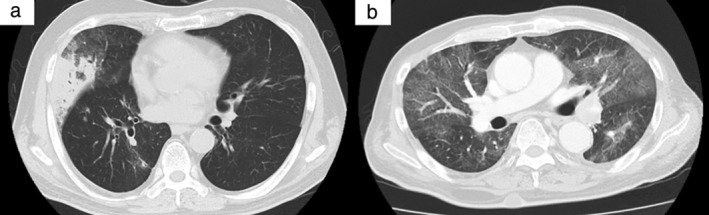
Computed tomography (CT) images of representative cases with severe grade checkpoint inhibitor pneumonitis. (a) Organizing pneumonia pattern. CT shows focal consolidations with surrounding ground‐glass opacities in right middle lobe. (b) Acute lung injury pattern. CT shows widespread ground‐glass opacities and consolidations in bilateral lungs.
